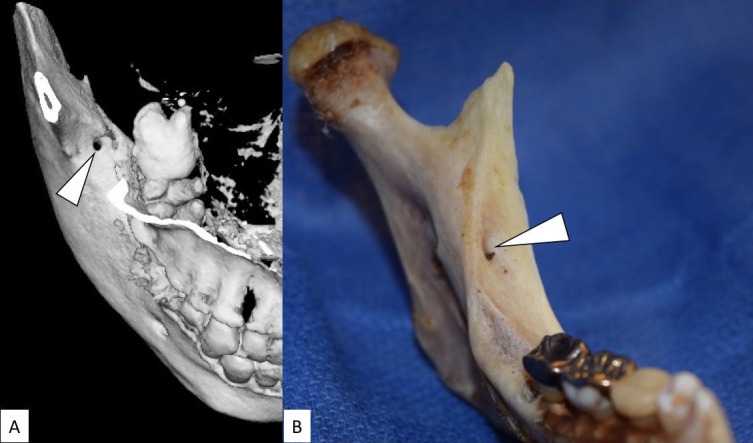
The retromolar foramen. The retromolar foramen (arrowhead) A: displays the right side in the computed tomography (superior view) B: displays the left side in the dry mandible (anterior-medial view).
